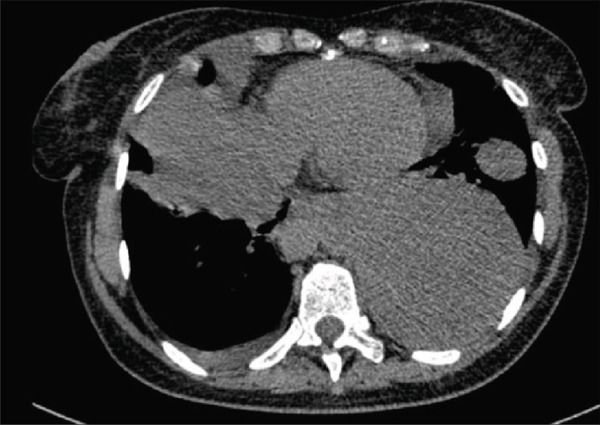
CT picture in the transverse plane. The finding at the time of disease progression (5/2020).
